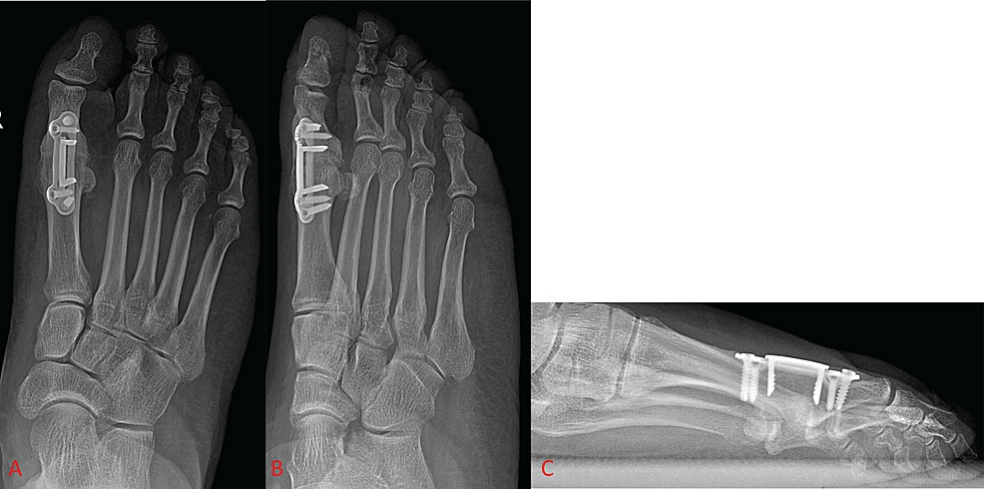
Foot X-ray shows hybrid dorsal locking plate with memory staple compression fixation of the first metatarsophalangeal joint. Figure 6A and Figure 6B show anterior-posterior and oblique views of the foot, respectively. Figure 6C shows a lateral view of the foot.
